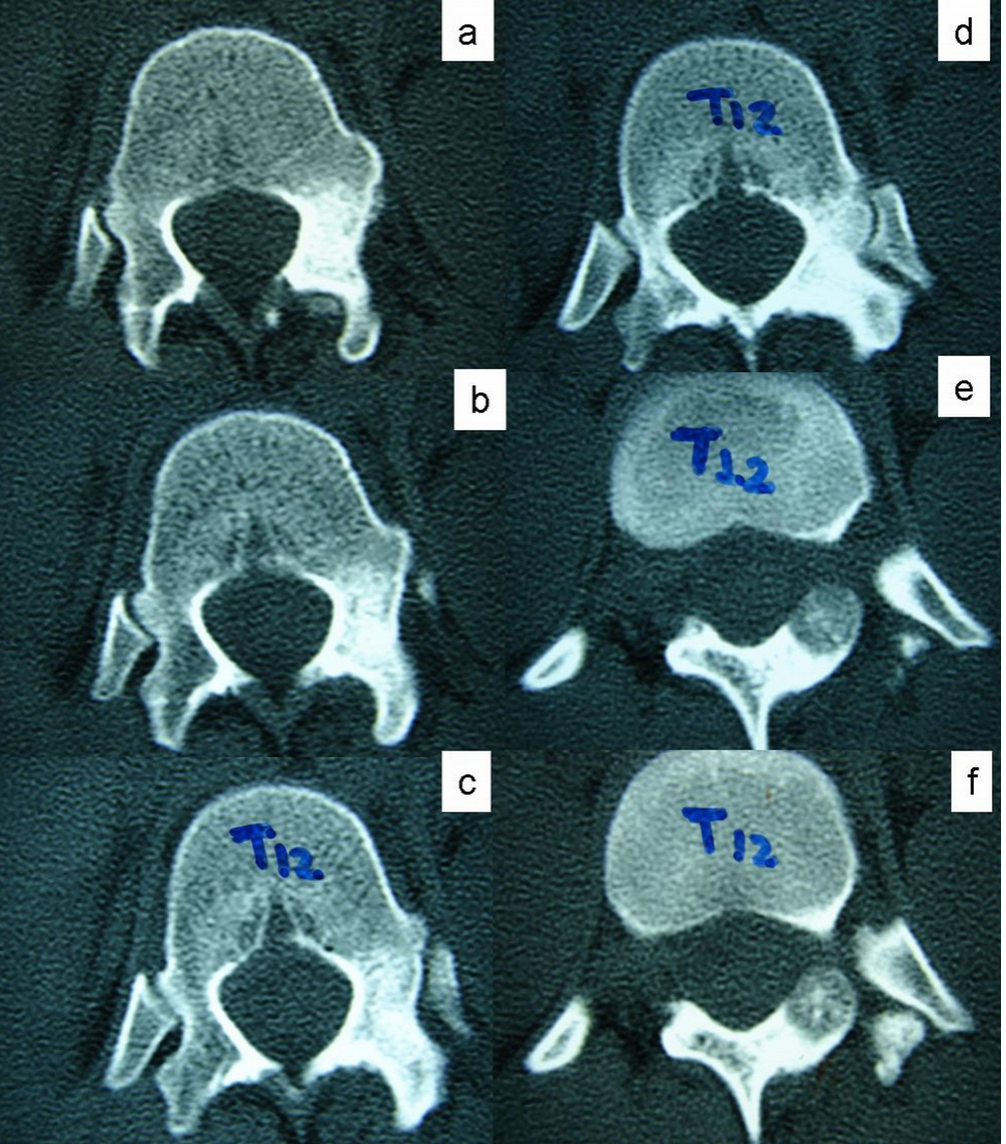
Consecutive CT slices of the T12 vertebra. The nidus can be seen at the pars interarticularis, with reactive sclerosis of the superior articular process and lamina and marrow oedema of the pedicle extending up to the vertebral body.
